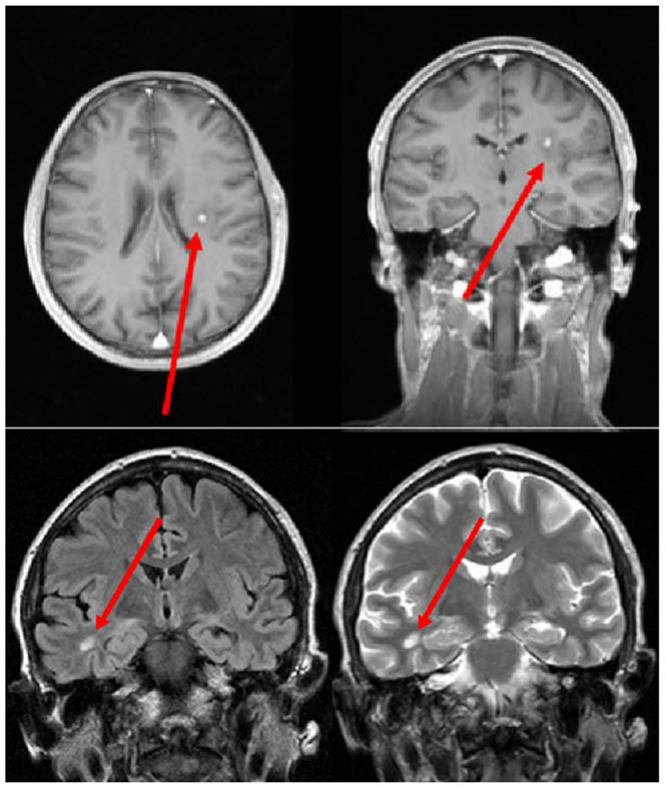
Case 8 (XP-C). Upper panel: T1-weighted conventional MRI post contrast in axial and coronal view. The arrow highlights a left-hemispheric contrast-enhanced lesion with perifocal edema within the frontoparietal white matter. Lower panel: Additional lesion in the temporal white matter presenting as a hyperintensity in coronal FLAIR (left) and T2-weighted (right) scans, marked by arrow. (For details, please cf. 3.2 Conventional MRI data.).
